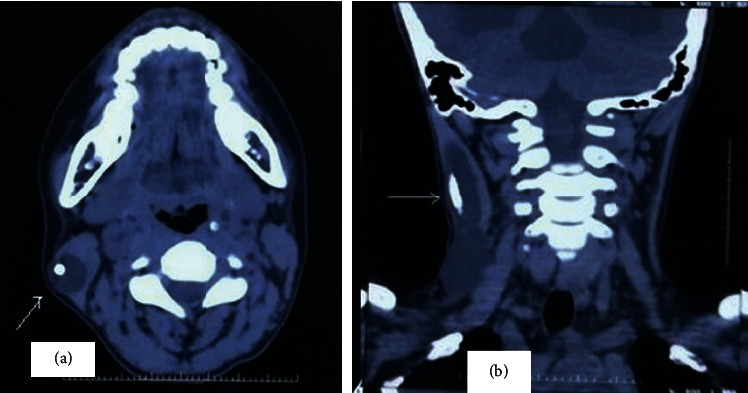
(a, b) Noncontrast computed tomogram of neck, coronal and axial sections showing the CSF pseudocyst involving the sternocleidomastoid on the right side with VP shunt in situ (white arrow).
